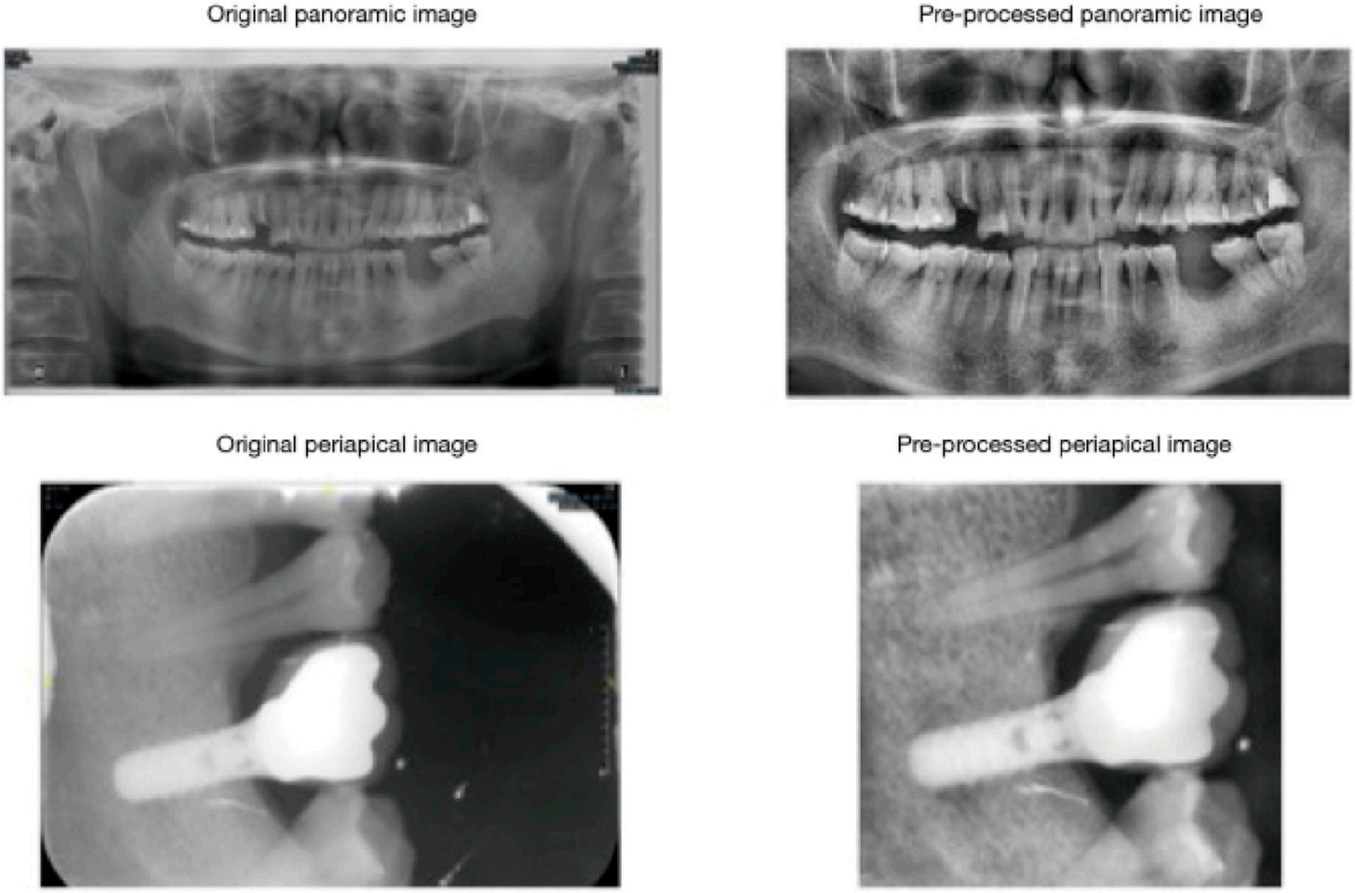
Cropped and histogram-equalized panoramic and periapical images demonstrate successful implant outcomes. Pre-processing steps, including cropping and histogram equalization, were applied to periapical and panoramic radiographs to optimize image contrast. Reproduced with permission from Zhang C. et al. (2023c).
